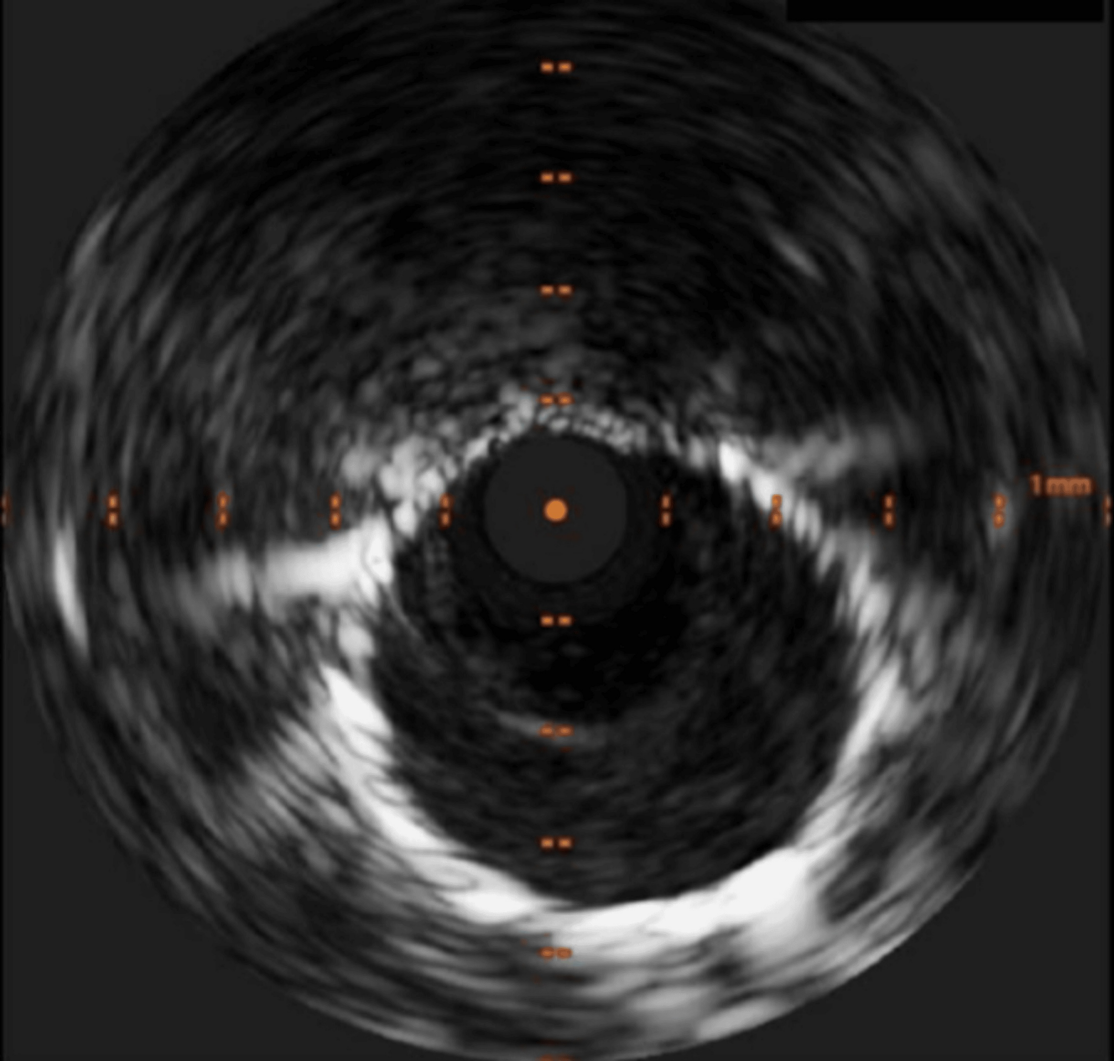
Intravascular ultrasound (IVUS) showing the covered stent
